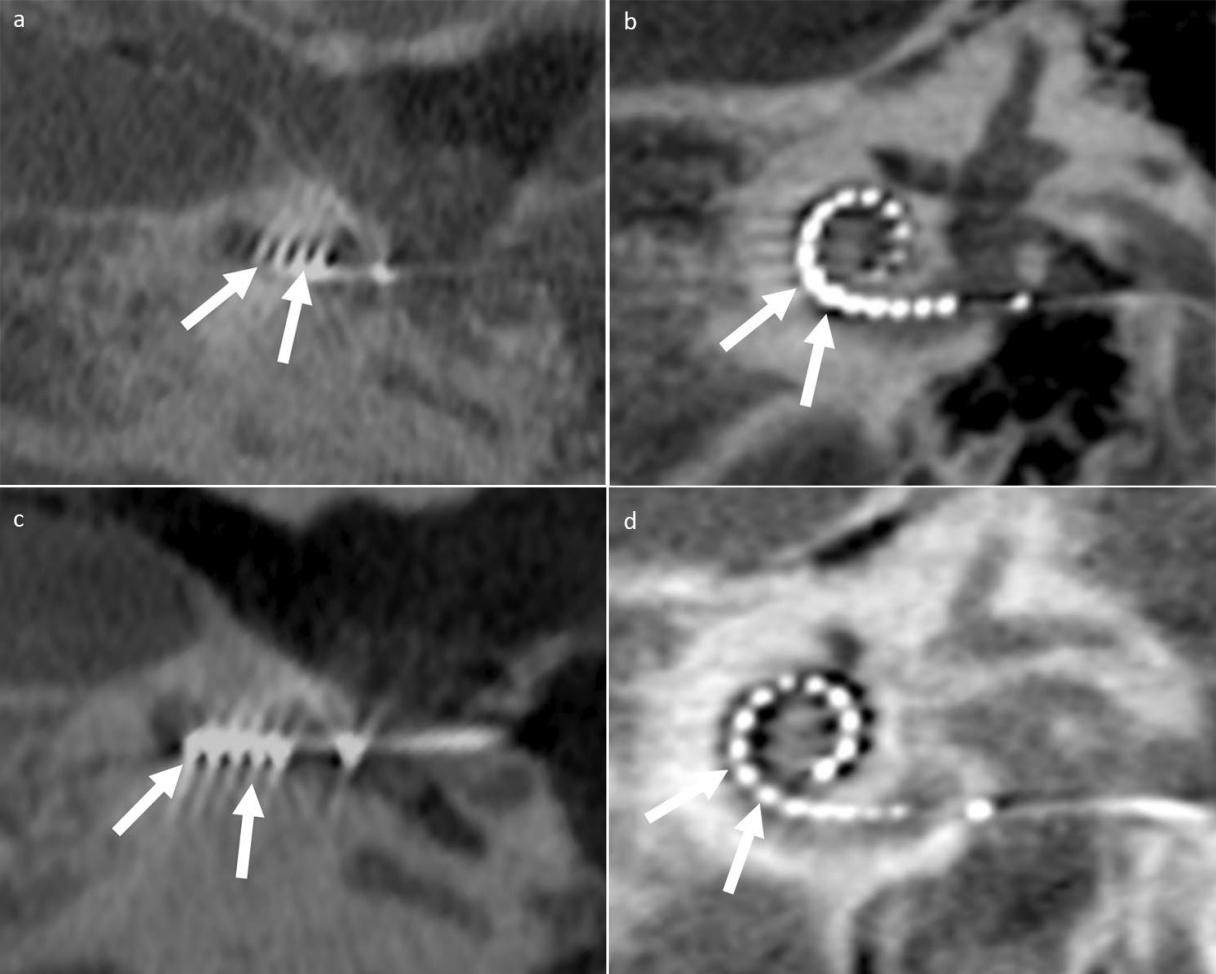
Representative 96-kV (A and C) versus 120-kV (B and D) CBCT axial views (basal turn of the cochlea is shown) and multiplanar reconstructions (Stenvers projection) with inserted Advanced Bionics HiFocus Mid Scala electrodes (Sonova Holding AG). (A) and (B) showing 96-kV images of a patient with bilateral congenital sensorineural hearing loss after left-sided CI surgery. (C) and (D) presenting a cochlea view and a Stenvers projection of a patient with unilateral left-sided surditas. 120-kV CBCT provides slightly better overall image quality with better delineation of the electrode contacts and depiction of the adjacent lateral wall of the cochlea compared to 96-kV (white arrows)
